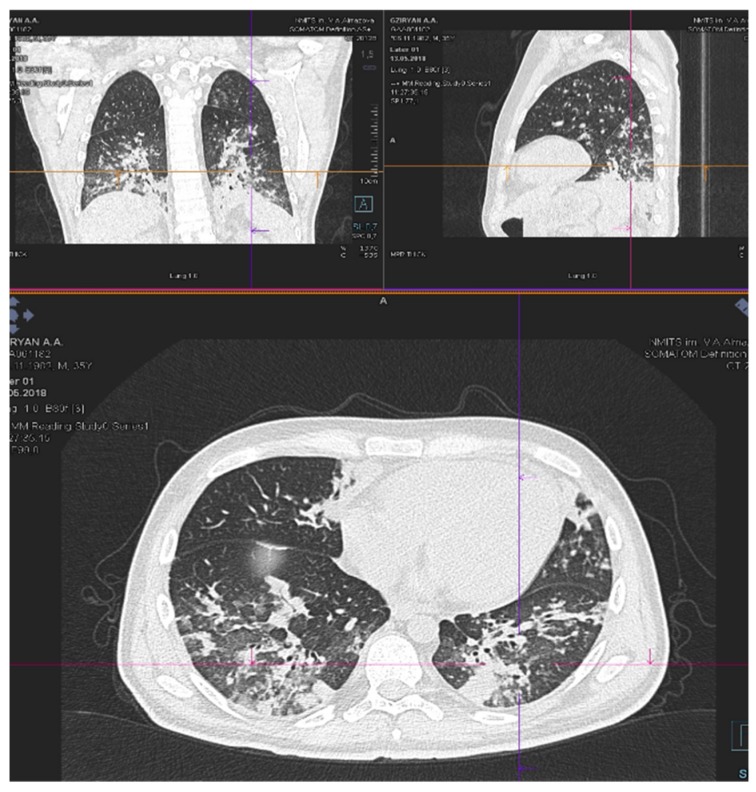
CT chest scan on day 18 of “FLAG”.
